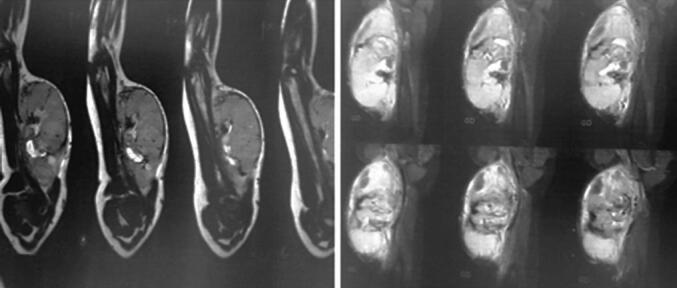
MRI of the left upper limb. T2-magnetic resonance imaging of the infantile fibrosarcoma demonstrating an ovoid mass with moderate hypersignal, traversed by vascular structures (a), which enhances in an intense and homogeneous manner after injection of gadolinium (b).
